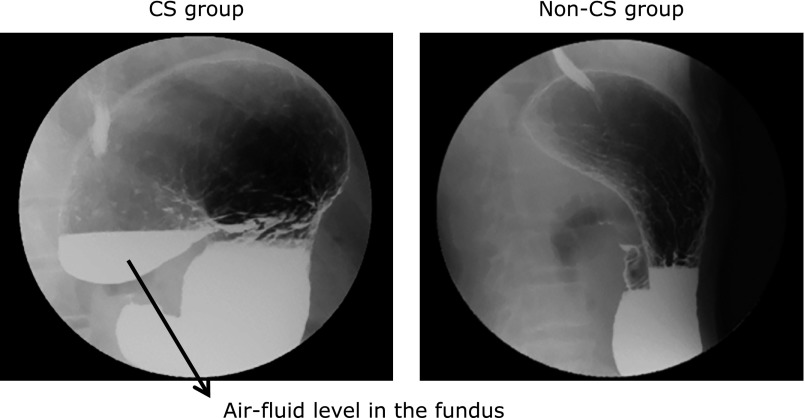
Classification of the stomach on X-ray films. CS was defined by detection of an air-fluid level in the fundus on an upright barium X-ray film. CS, cascade stomach; non-CS, non-cascade stomach.
